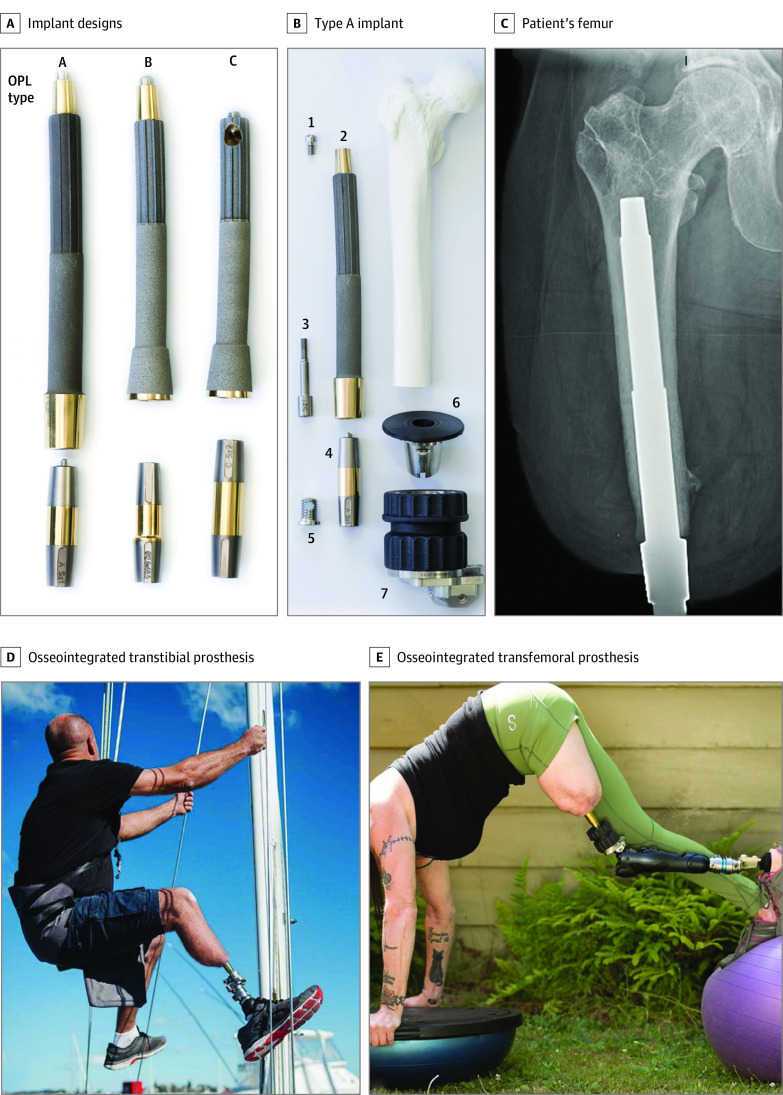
Presentation of Transcutaneous Osseointegration Post Amputation (TOPA) A, Photograph of common osseointegrated prosthetic limb (OPL [Permedica]) implant designs. The top portion is made of titanium with a textured intramedullary portion and smooth transcutaneous portion and is implanted into the patient’s residual limb. The dual cone below the implant is a connector to the external prosthetic limb. B, Exploded view of a type A implant with the components arranged at approximately the proximal-distal levels in which they would be once assembled and implanted in a patient who had undergone a femoral amputation. 1, Proximal cap screw; 2, OPL body; 3, safety screw; 4, dual cone transcutaneous adapter; 5, prosthesis adapter screw; 6, proximal connector; and 7, prosthetic connector. C, Anterior-posterior radiograph of a TOPA implant in a patient’s femur. The textured portion is intramedullary; the smooth portion is transcutaneous. D and E, Examples of activities that a patient with an osseointegrated transtibial (D) or transfemoral (E) implant can achieve with a skeletally connected prosthesis.
